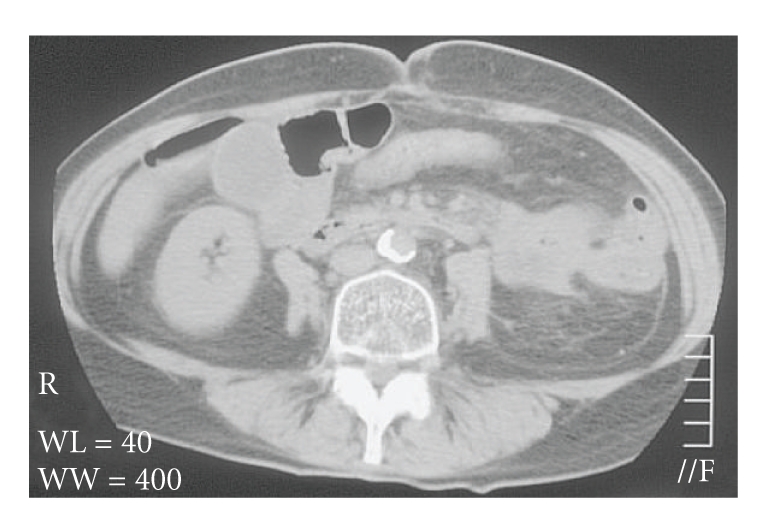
CT of the abdomen 2 months after palliative radiotherapy showing absence of residual tumor in the jejunum.
